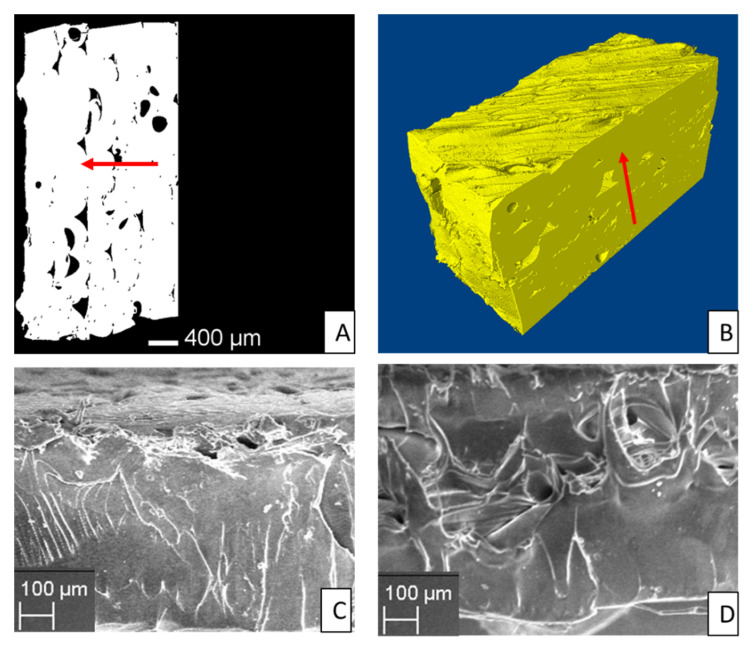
Micro CT analysis; segmented cross section (A) and 3D model from CT (B). Red arrows indicate the deposition direction. SEM images of the cross section of PET_3D sample (C,D).
